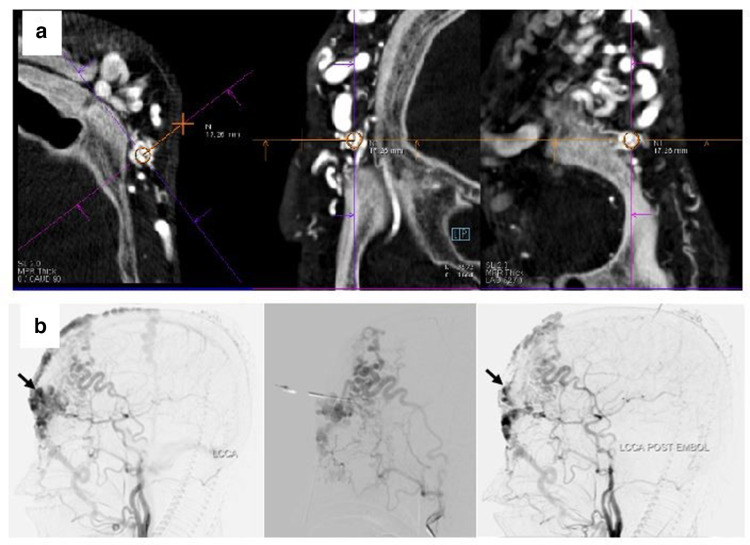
Case 2, AVM of the left frontotemporoparietal and superior palpebra. (a) CBCT-A in axial, sagittal, and coronal views demonstrating the projection of the virtual needle targeting the left superficial temporal artery. (b) DSA images showing (left) multiple nidus at the left frontotemporoparietal dan superior palpebra region, (middle) needle insertion to the target vessel, and (right) nidus occlusion at the left frontal region following glue administration. AVM, arteriovenous malformation; CBCT-A, cone beam computed tomography angiography; DSA, digital subtraction angiography.
